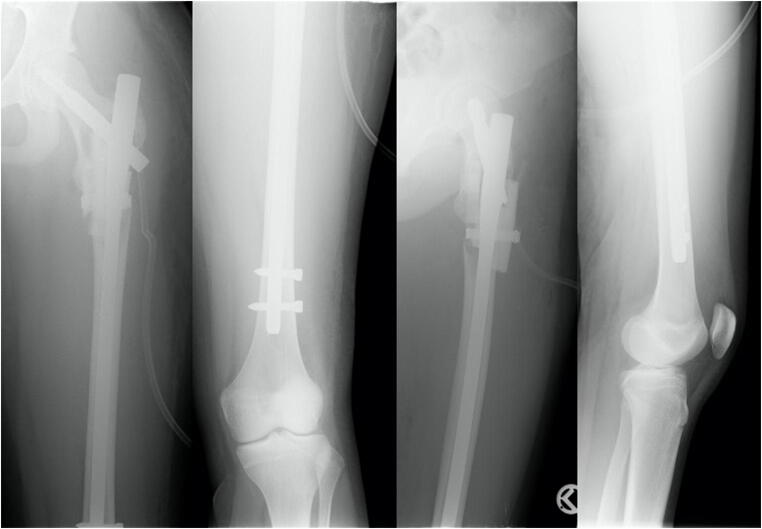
Anterior-posterior and axial X-ray of the left femur after surgical stabilization with a long gamma nail and a cerclage
